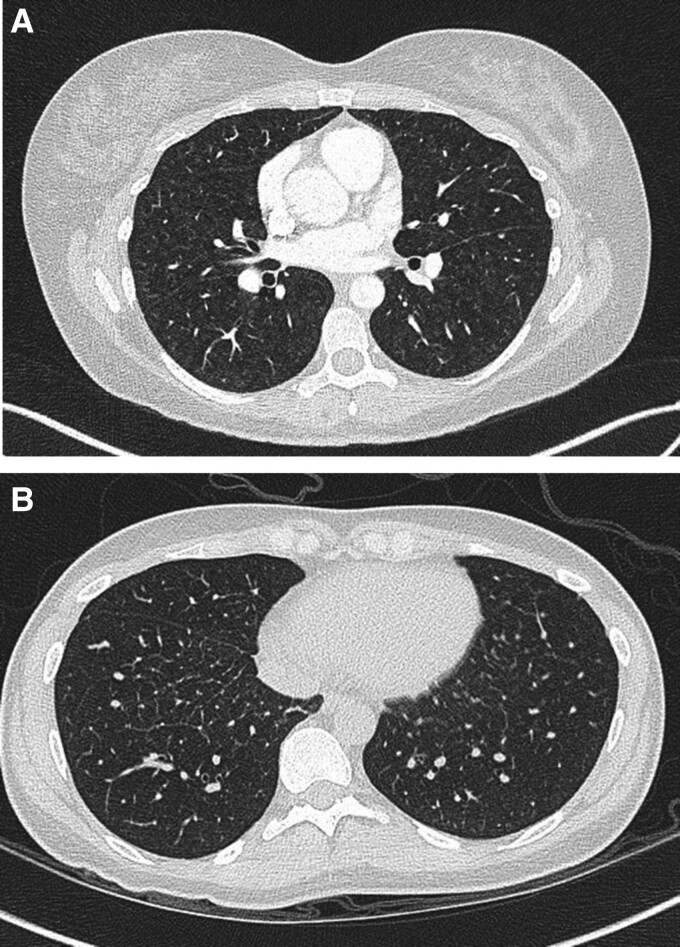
(*A*) High-resolution computed tomography of Patient 1 at diagnosis showing soft ground glass nodularity and subtle interlobular septal lines. (*B*) High-resolution computed tomography of Patient 2 at follow-up showing interlobular septal thickening and mild parenchymal lung nodularity.
